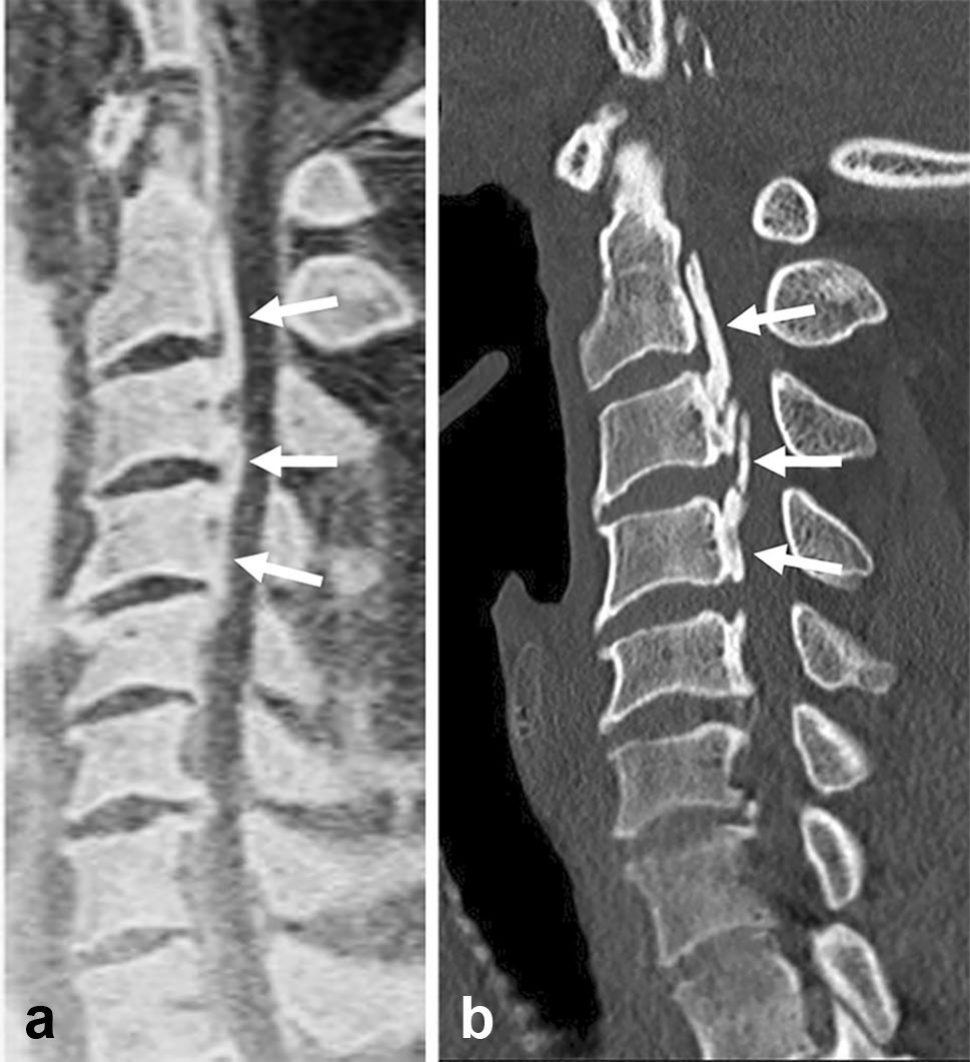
Ossification of the posterior longitudinal ligament. In diagnosing ossification of the posterior longitudinal ligament (OPLL), it is imperative to precisely assess the degree of ossification that causes spinal cord compression. Sagittal MR bone image a shows segmented OPLL at C2-C4 extending partially to C5 (arrows) in a 43-year-old male patient. Sagittal CT image b confirms the OPLL (arrows)
